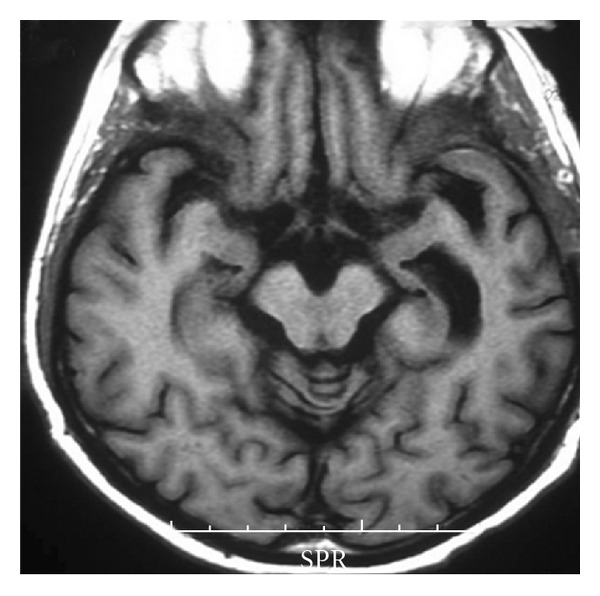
Axial T1-weighted contrast image shows almost complete resolution of the temporal lobe lesion with mild dilatation of the left temporal horn.
